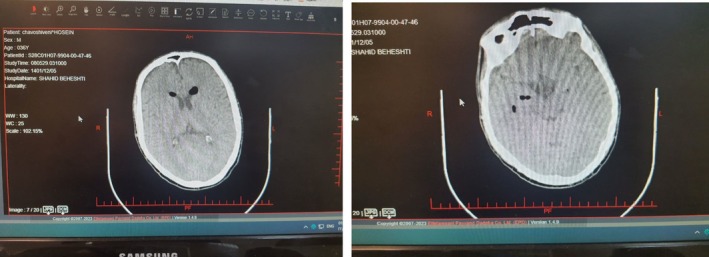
Axial noncontrast computed tomography (CT) images of the brain in a 37‐year‐old male patient who developed pneumocephalus following epidural anesthesia for hip joint replacement. Multiple small intracranial air foci are visible within the frontal region and subarachnoid space, which is consistent with pneumocephalus. No evidence of intracranial hemorrhage or midline shift were observed.
